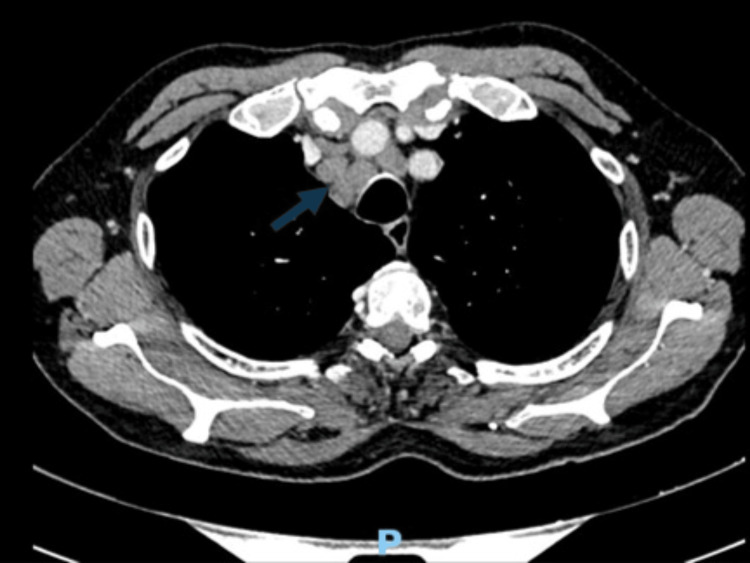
Thoracic CT showing multiple mediastinal lymphadenopathies, including a right paratracheal node measuring approximately 20 mm.
